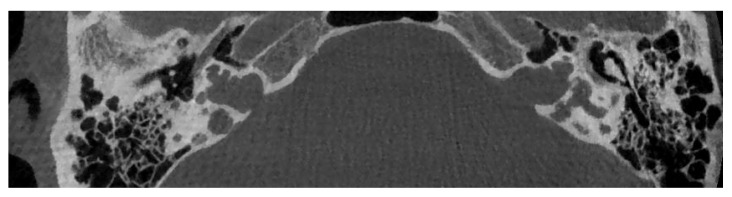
CT imaging of a bilateral incomplete partition type 3. The cochlear structures appear to be symmetrically altered, with the typical appearance of an empty cochlea, without a clear fundus of the internal auditory canal.
